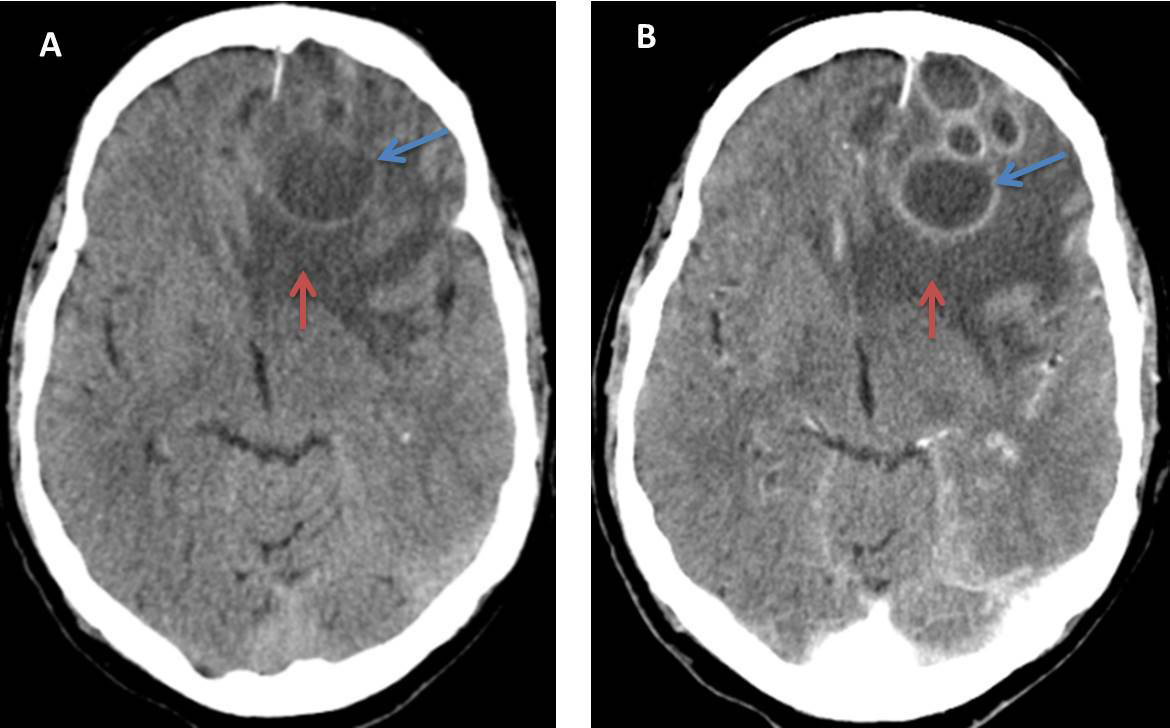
Axial scans cerebral CT without intravenous contrast (A) and after administration of intravenous contrast (B). Several frontal left hypodense nodular lesions (red arrow in A) with “ring” enhancement (red arrow in B) are visualized. They associate perilesional edema (blue arrows in A, B).
